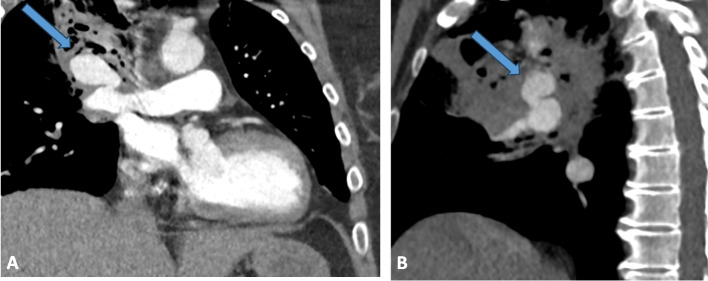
CT scan revealing a proximal false aneurysm of the right pulmonary artery (blue arrow) in coronal section A and sagittal section B
